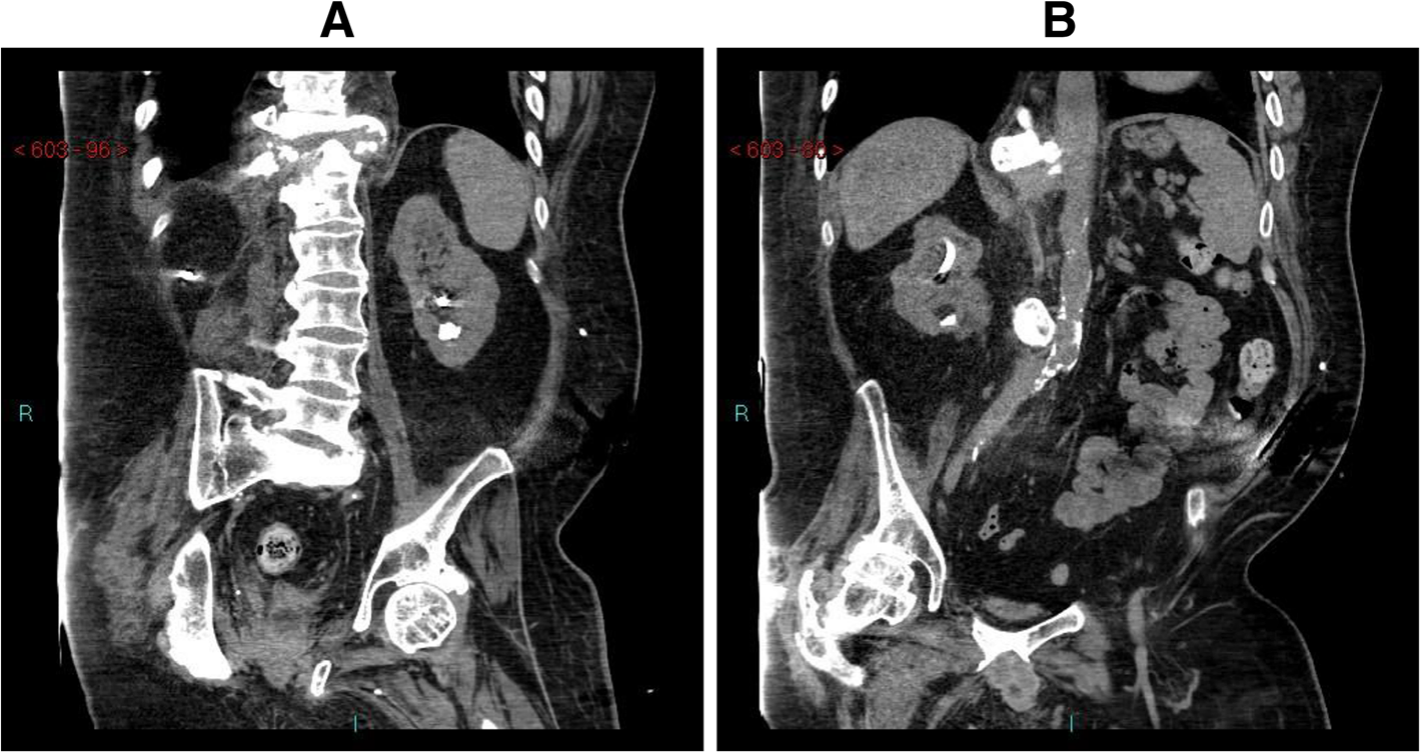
Case 1: Computed Tomography of kidneys, coronal section. (A) Left kidney. Calculus in lower calyx; left nephrostomy in place; renal outline is irregular due to cortical scarring. (B) Right kidney: stone in lower pole; right nephrostomy in place; cortical margin is irregular. Calcification of aorta is seen.
